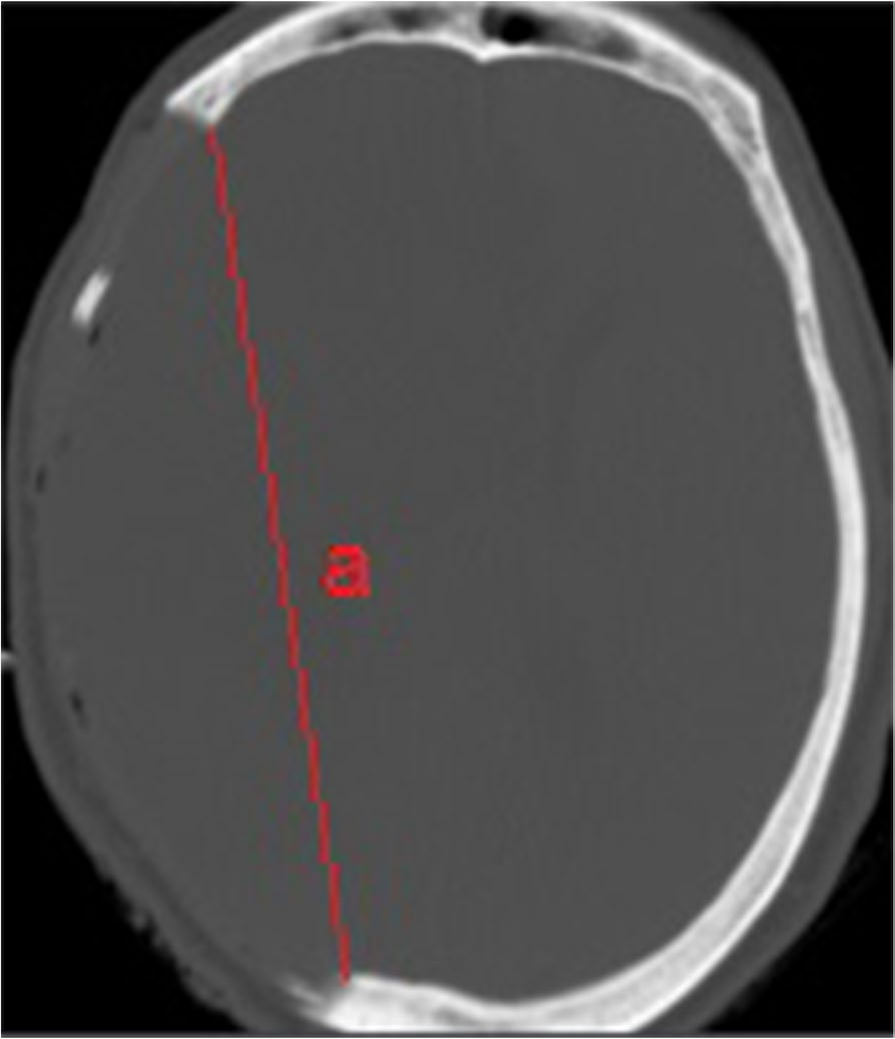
CT scan of a brain after craniectomy. a, diameter was measured by length of a line connecting inner tables of the cranium
